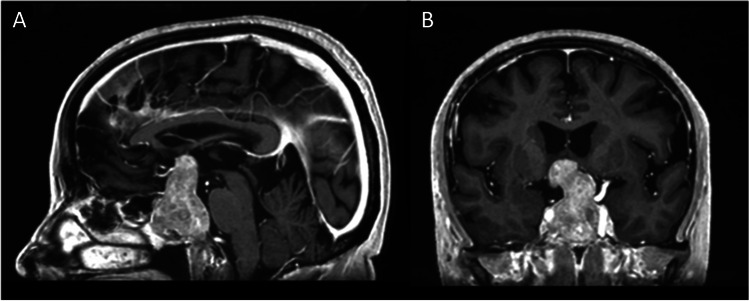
Preoperative MRI patient 1 showing a macroadenoma in A sagittal view and B coronal view
